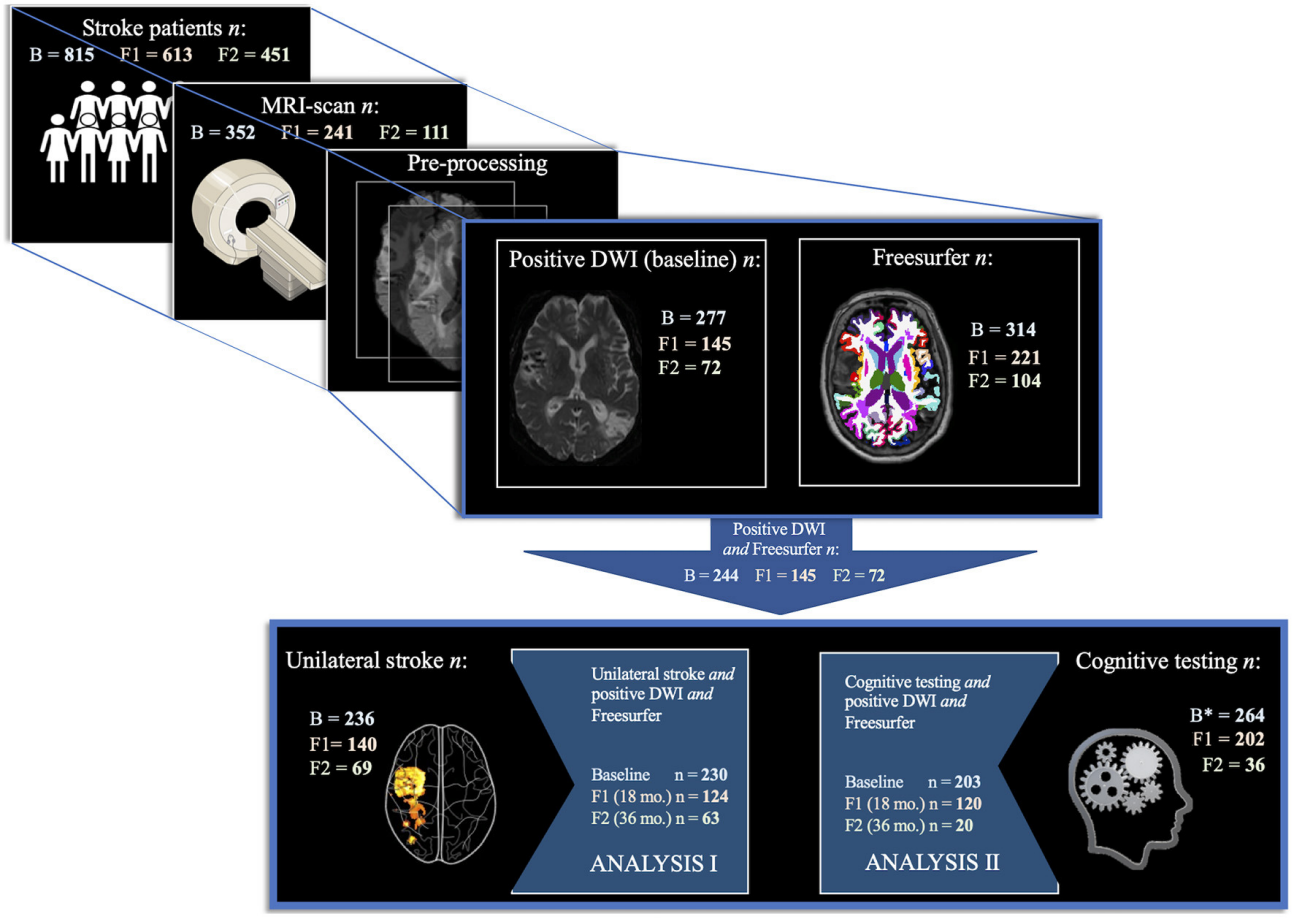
Study population selection. Study population selection by analysis per timepoint. The figure depicts the inclusion process from 815 Nor-COAST participants at baseline, to 352 included in the MRI sub-study, whereupon 277 of these also had the stroke lesion visible on DWI, and 314 had successful Freesurfer anatomical segmentation, resulting in a final study sample of 244 at baseline. Out of these, 230 had a unilateral stroke and was used in analysis I. Out of the final study sample, 203 had cognitive testing at 3 months and was used in analysis II. B, Baseline. B^*^, Baseline (3 months). F1, Follow-up 1 (18 months). F2, Follow-up 2 (36 months).
